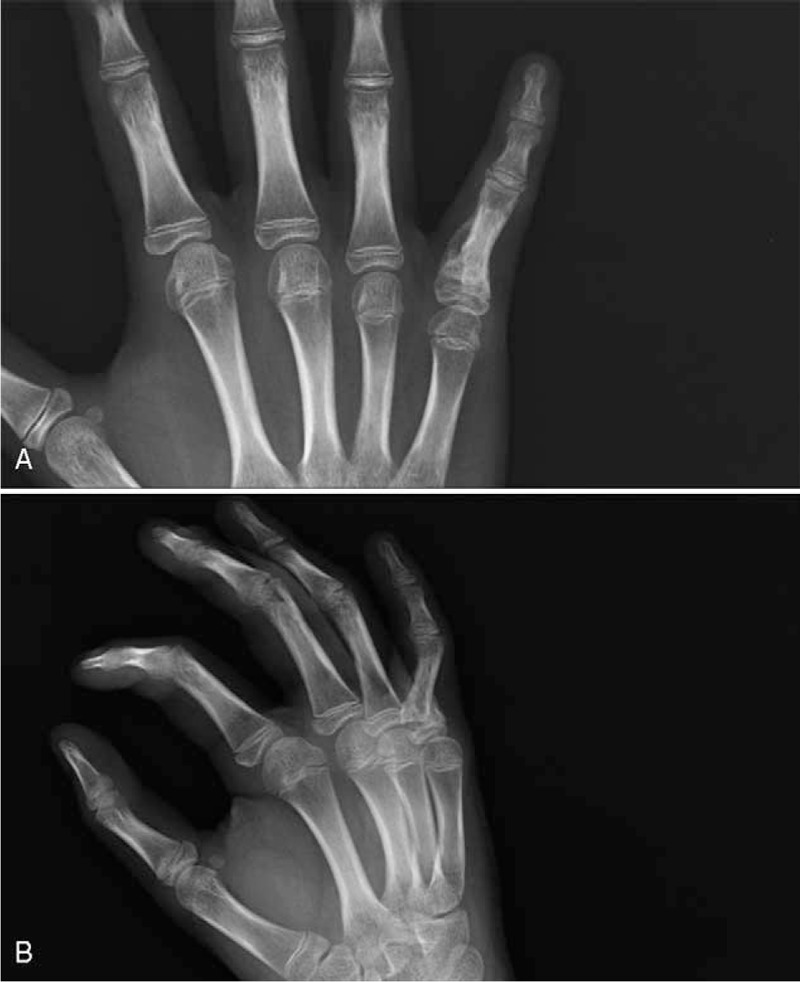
A–B. Preoperative AP (A) and oblique (B) radiographs demonstrate a Salter–Harris type II fracture of the small finger proximal phalanx base and volar angulation with callus formation.
